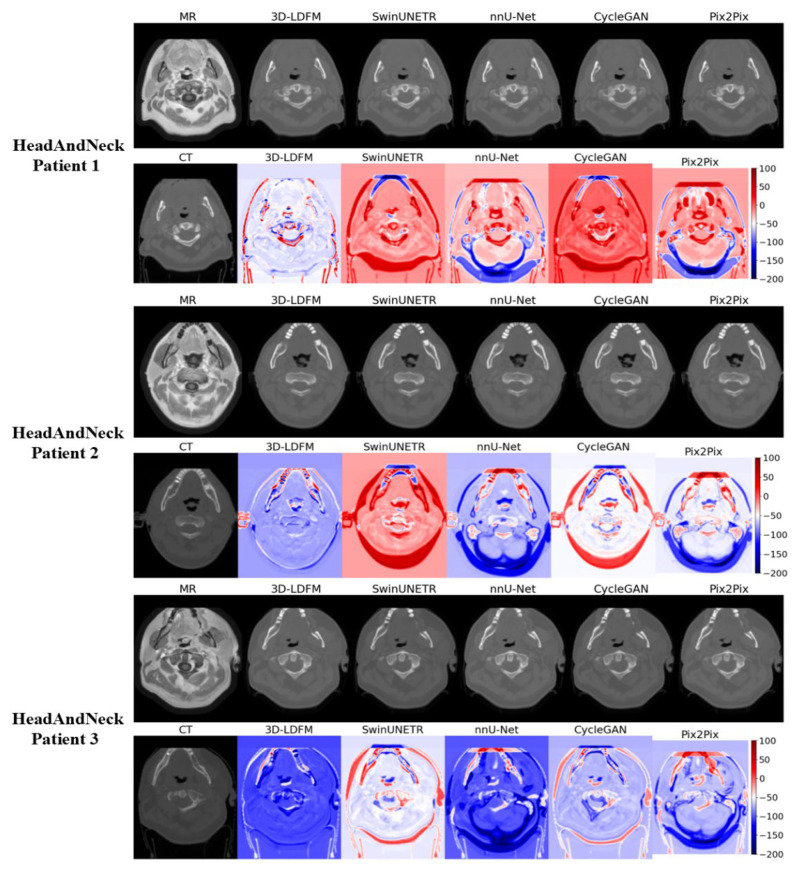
Head-and-Neck Slices within the Brain Volume. Qualitative comparison and error maps for sCT generation on inferior slices of the brain MRI/CT volumes that include head-and-neck anatomy.
